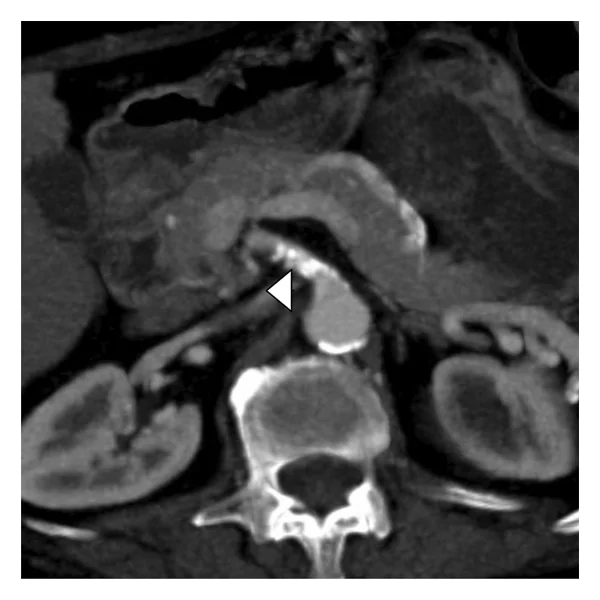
Axial contrast–enhanced abdominal CT image demonstrating calcific plaques located at the origin of the SMA. (Arrowhead) The calcifications appear as bright, dense areas, indicating the presence of atherosclerotic plaques at the point where the SMA originates from the abdominal aorta. This calcific buildup can contribute to narrowing of the artery and reduce blood flow to the intestines.
